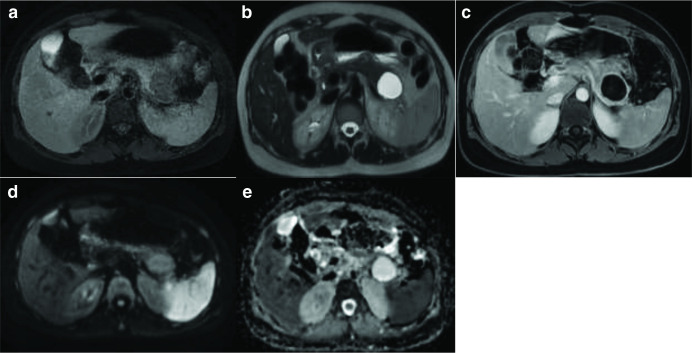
MRI axial sequences of the upper abdomen. A. T1 FATSAT showing a hypointense
